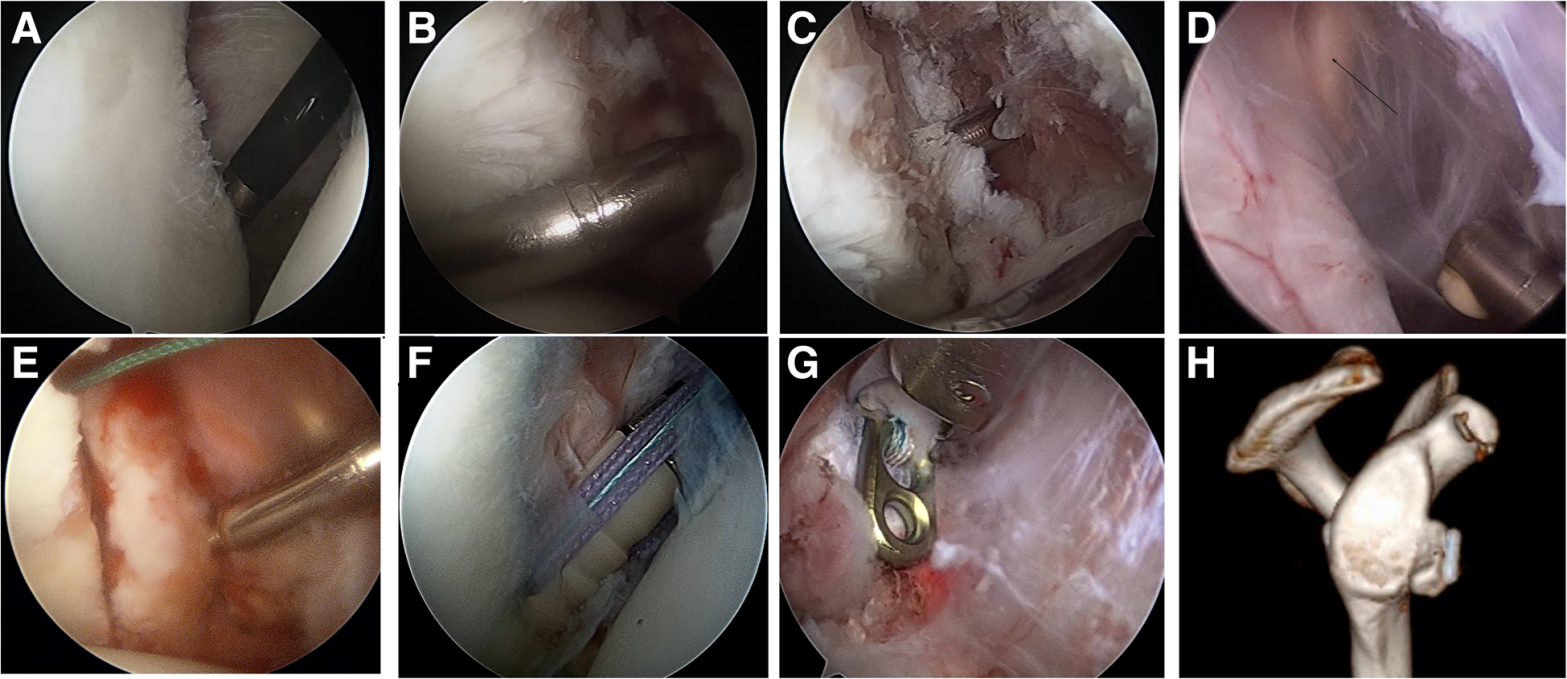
The surgical steps for MSB Latarjet procedure. a The glenoid bone defect was exposed. A marker was made on the anterior glenoid edge at half past 3 o’clock for location by the radiofrequency. b Debridement was performed on the glenoid and the switching stick was used to locate the accurate position for en-face graft fixation. c A tunnel with a diameter of 4.5 mm was drilled into the glenoid at the pre-prepared location with the guidance of custom-made guiding instrument. d The axillary nerve was exposed in case of injury. e High-strength sutures in the proximal tunnel of the bone block were passed into the tunnel of the glenoid. Then the coracoid block was pulled into the shoulder joint using the three sutures and firmly adhered to the glenoid. f The other suture in the distal tunnel was fixed together with a knotless anchor to the glenoid at half past 3 o’clock to prevent coracoid block rotation. g The suture button’s position was adjusted to prevent itself from the impingement of the humeral head. h The en-face view of the graft position from the CT scan at postoperative day 1
